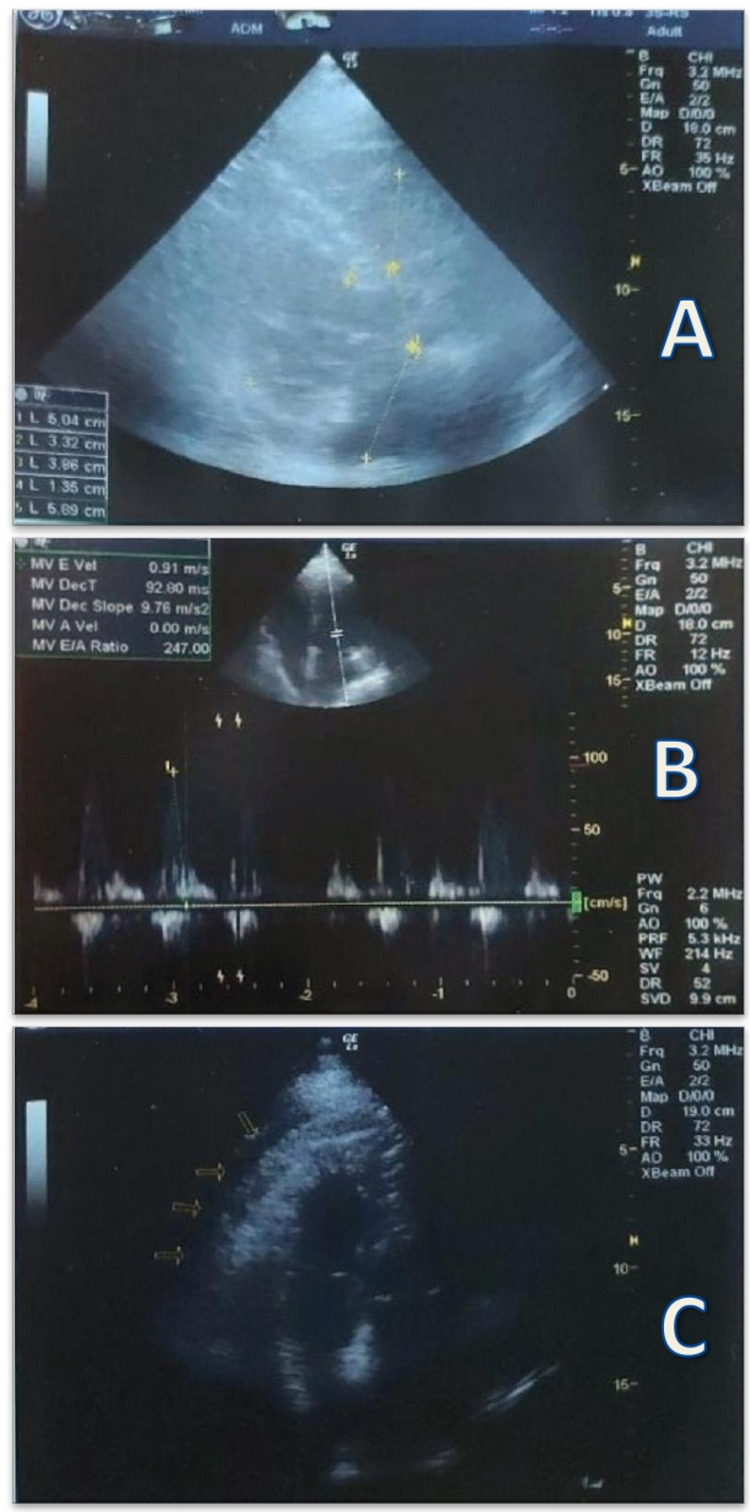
Echocardiography revealed an LV-ejection fraction of 50%-55% with grade III left ventricular diastolic dysfunction, biatrial and biventricular dilatation, a mild pericardial effusion, and a moderate septal hypertrophy (A) Parasternal long-axis imaging depicting biventricular and left atrial dilatation and moderate septal hypertrophy. (B) Pulsed wave Doppler study of transmitral inflow in atrial fibrillation, indicating early transmitral flow deceleration time (DT). E represents peak LV early diastolic filling velocity. Peak E velocity and DT vary depending on cardiac cycle length. Grade III left ventricular diastolic dysfunction. (C) Apical four-chamber view depicting a mild pericardial effusion.
